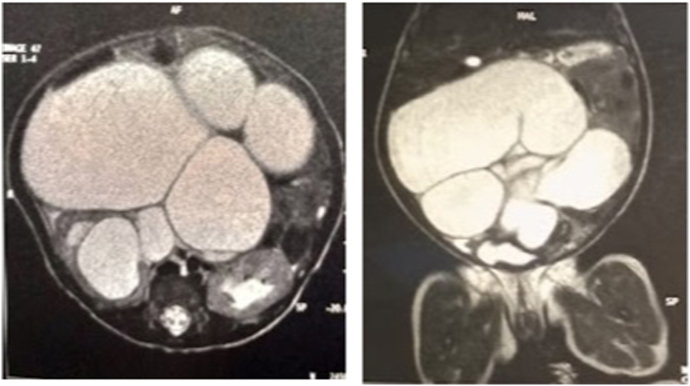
MRI cross-section in 1–1 showing bilateral hydronephrosis and right geant megaureter and ipsilateral renal parenchyma thinning. Coronal section in^1^,^2^ showing an important ureter dilatation.
